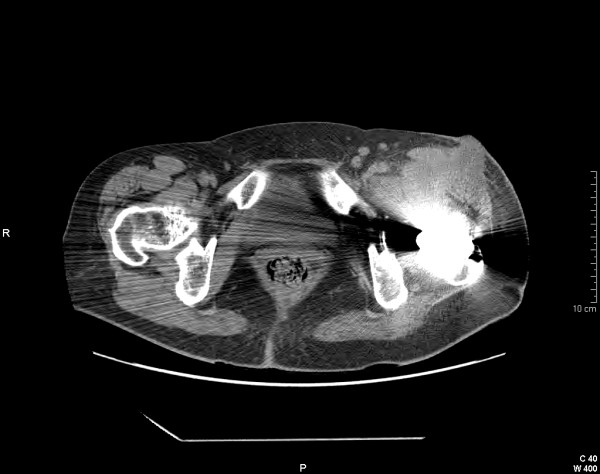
A computed tomography scan image of hip arthroplasty. Evidence of a periprosthetic abscess with diffusion to the left psoas muscle and cutaneous fistula is shown.
